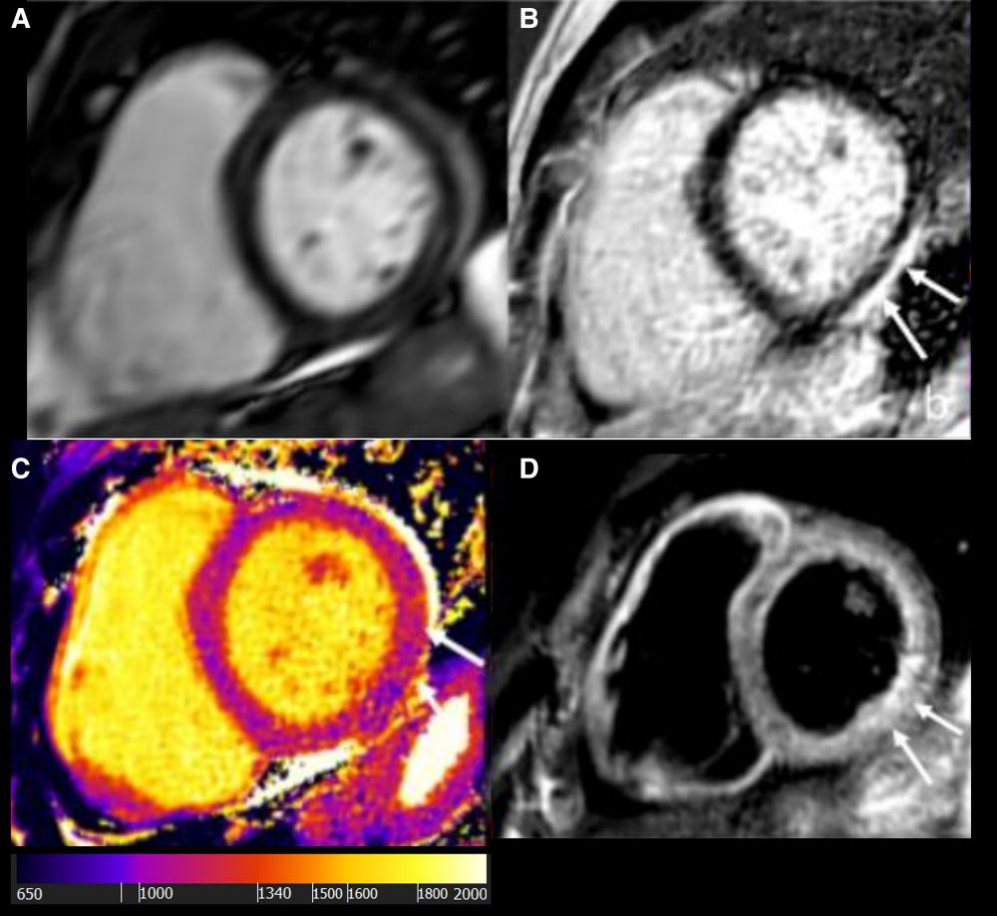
Cardiovascular magnetic resonance imaging basal short axis. (*A*) Steady-state free precession image, normal wall thickness and a small pericardial effusion. (*B*) Late Gadolinium Enhancement images with sub-epicardial enhancement in the basal inferolateral segment (arrows). (*C*) Native T1 map demonstrating severely increased T1 values in the basal inferolateral segment 1151 ms (normal 1013 ± 35 ms) (arrows). (*D*) T2 weighted black blood images demonstrating increased signal in the basal inferolateral segment suggestive of myocardial oedema (arrows).
